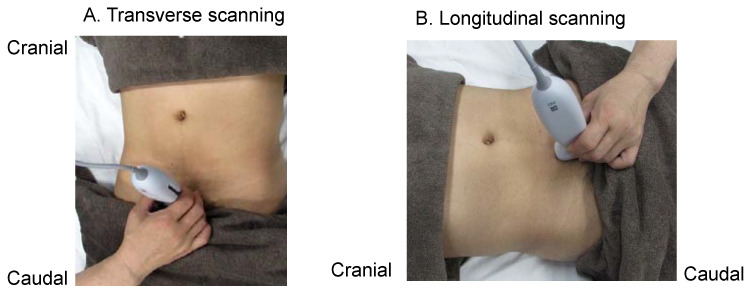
How to apply the ultrasound probe. The probe is placed at the superior margin of the pubis for both transverse scanning (A) and longitudinal scanning (B). In both techniques, the ultrasound beam is tilted caudally by 10–30 degrees to visualise the bladder. The bladder is used as an acoustic window, with the rectum being visualised deeper than the bladder.
